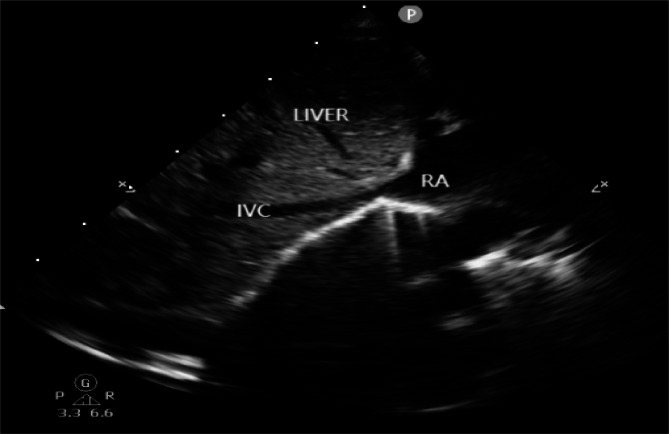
2-D ultrasound showing IVC entering the right atrium
